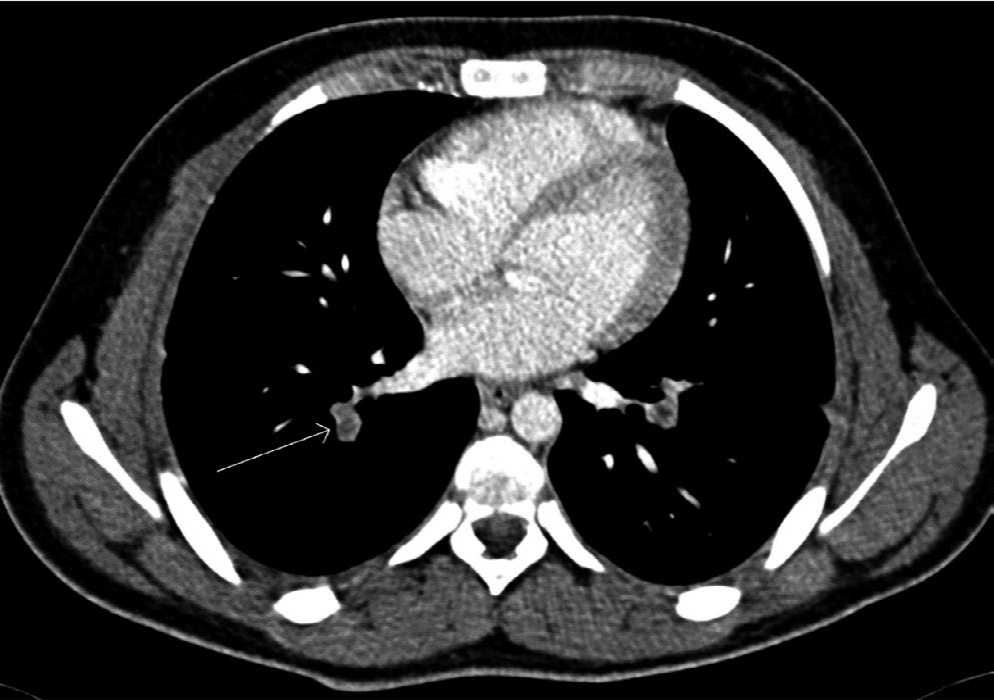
CT chest showed right side pulmonary embolism
